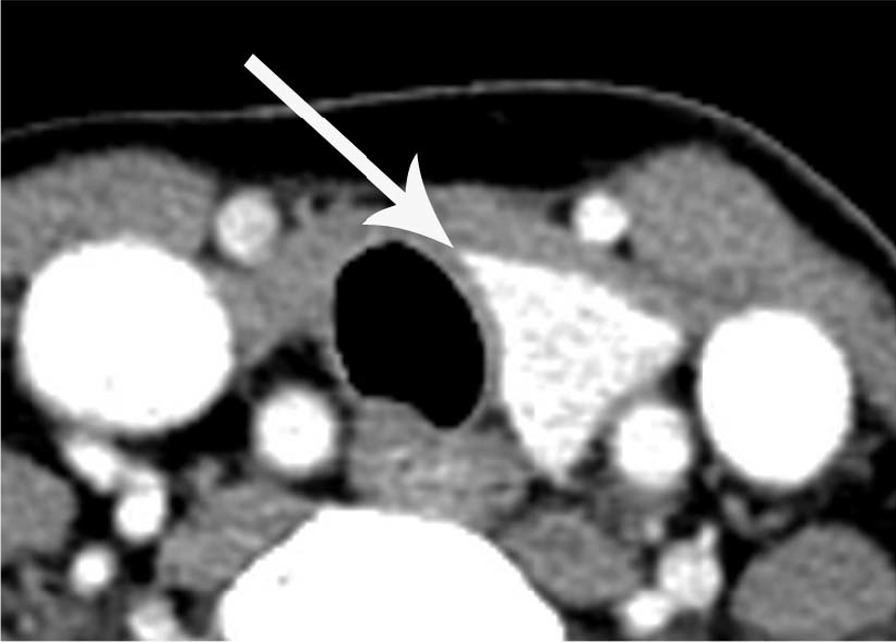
A 65-year-old woman with right thyroid hemiagenesis (case 5). Axial contrast-enhanced CT scan shows the absence of isthmus and sharp edge of medial end of remnant thyroid gland (arrow). The angle of the medial end of remnant thyroid gland was measured as + 29°
